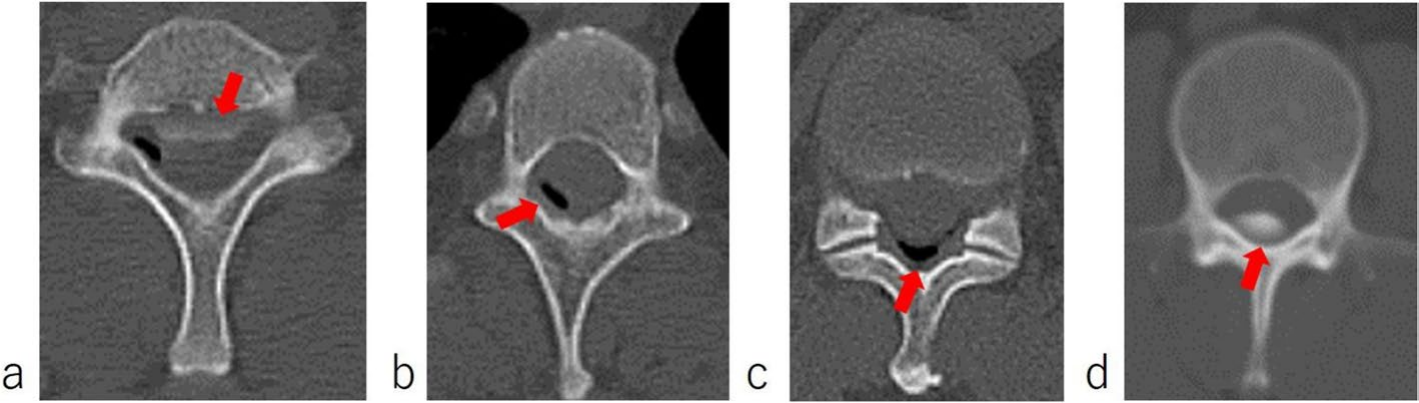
Post procedural CT results (a) Contrast agent accumulation in the ventral epidural space at the level of the second thoracic vertebra(arrow). b Air retention in the epidural space at the level of the third thoracic vertebra (arrow). c Air retention in the epidural space at the level of the 12th thoracic vertebra (arrow). d Contrast agent accumulation in the subdural space below the level of the 12th thoracic vertebra (arrow)
